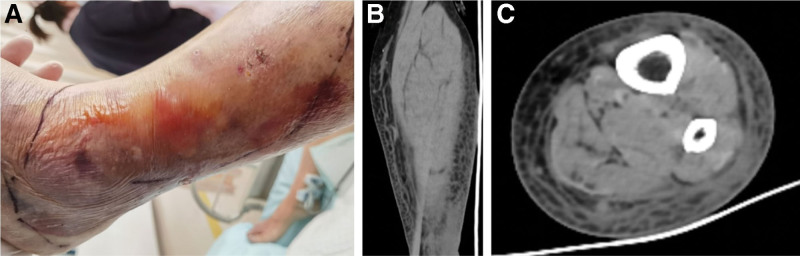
(A) At the time of intensive care unit admission, the patient’s lower left leg exhibited redness, swelling, ulcers, blisters, and pus spots on the lateral surface. (B, C) CT of the left leg showed soft tissue swelling in the middle and lower segments, blurred subcutaneous fat space, grid changes, and significantly thickened deep fascia. CT = computed tomography.
